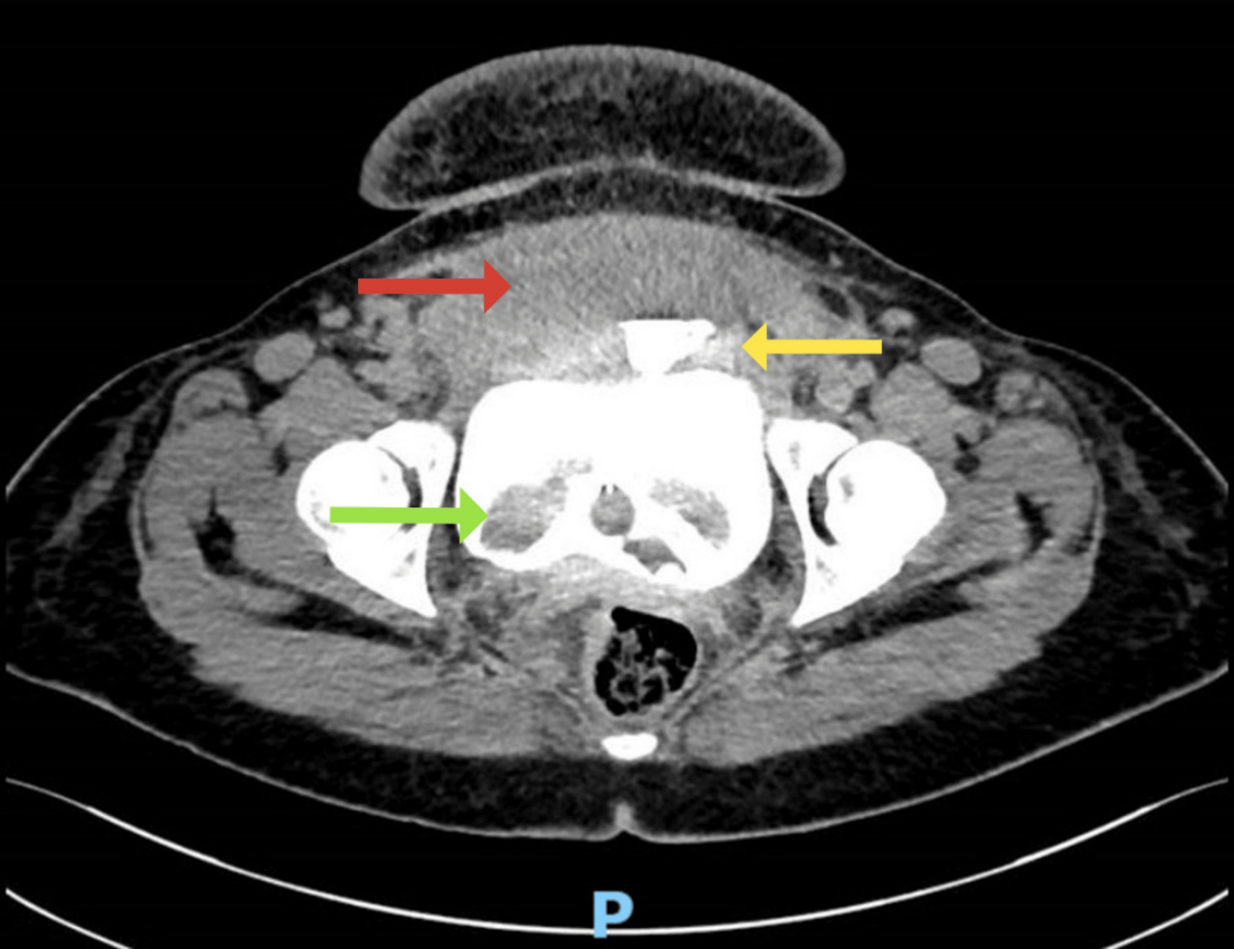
Axial view of the initial CT cystogram showing extravasation of contrast that is spreading above the urinary bladder (yellow arrow). There is significant pelvic fluid along the anterosuperior aspect of the bladder beneath the anterior abdominal wall measuring 111 x 88 x 38 mm with contrast leaking through this collection (red arrow). Urinary bladder shows catheter and blood clot within its lumen (green arrow).
